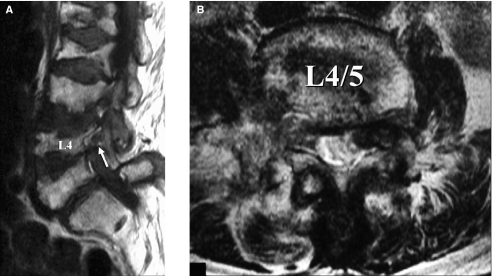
Magnetic resonance imaging (MRI). A: In the sagittal section (right L4/5 foramen; T1-weighted image), foraminal stenosis is not obvious (arrow). B: In the transverse section (L4/5 foramen; T2-weighted image) as well, foraminal stenosis is not evident.
